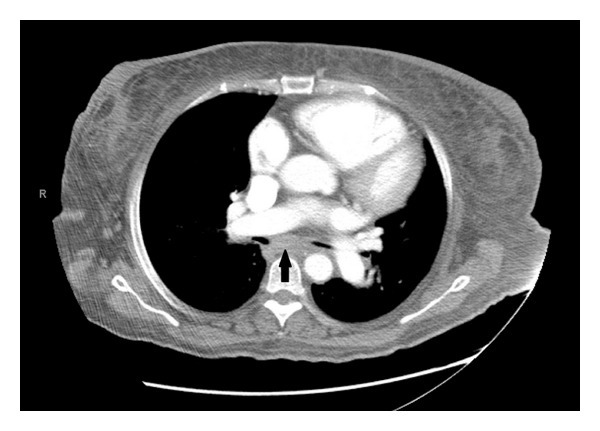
Chest CT showing mediastinal lymphadenopathy (arrow).
